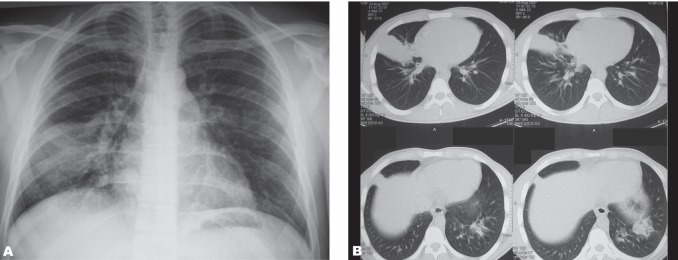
A 22-year-old male patient with fire eater’s pneumonia.( A. A relatively homogeneous density was determined without eliminating the heart and diaphragm contours in his chest radiography. B. Consolidation areas covering the lateral segment of the right middle lobe together with small nodules in the medial segment of the middle lobe and lateral basal segments of the lower lobe. Bronchopneumonic consolidation areas in the left lower lobe are detected in thoracic CT.)
